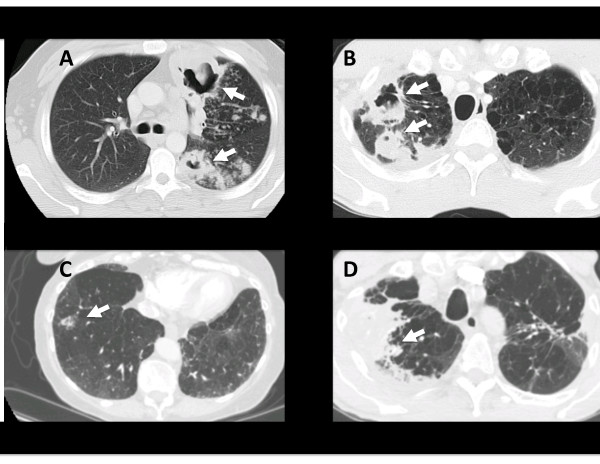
Representative CT images for mycobacterial infections. Shown are images for (A) *M. tuberculosis*, with cavities (arrows), in a 35 y.o. foreign-born individual with no predisposing risk factor for NTM, (B) *M. kansasii*, with cavity (arrow), in a 51 y.o. male with COPD, (C) *M. avium*, with nodule (arrow), in a 70 y.o. female with COPD, and (D) *M. xenopi*, with 7 cm peripheral wedge shaped consolidation (arrow) with air cavities, in 66 y.o. male with COPD.
